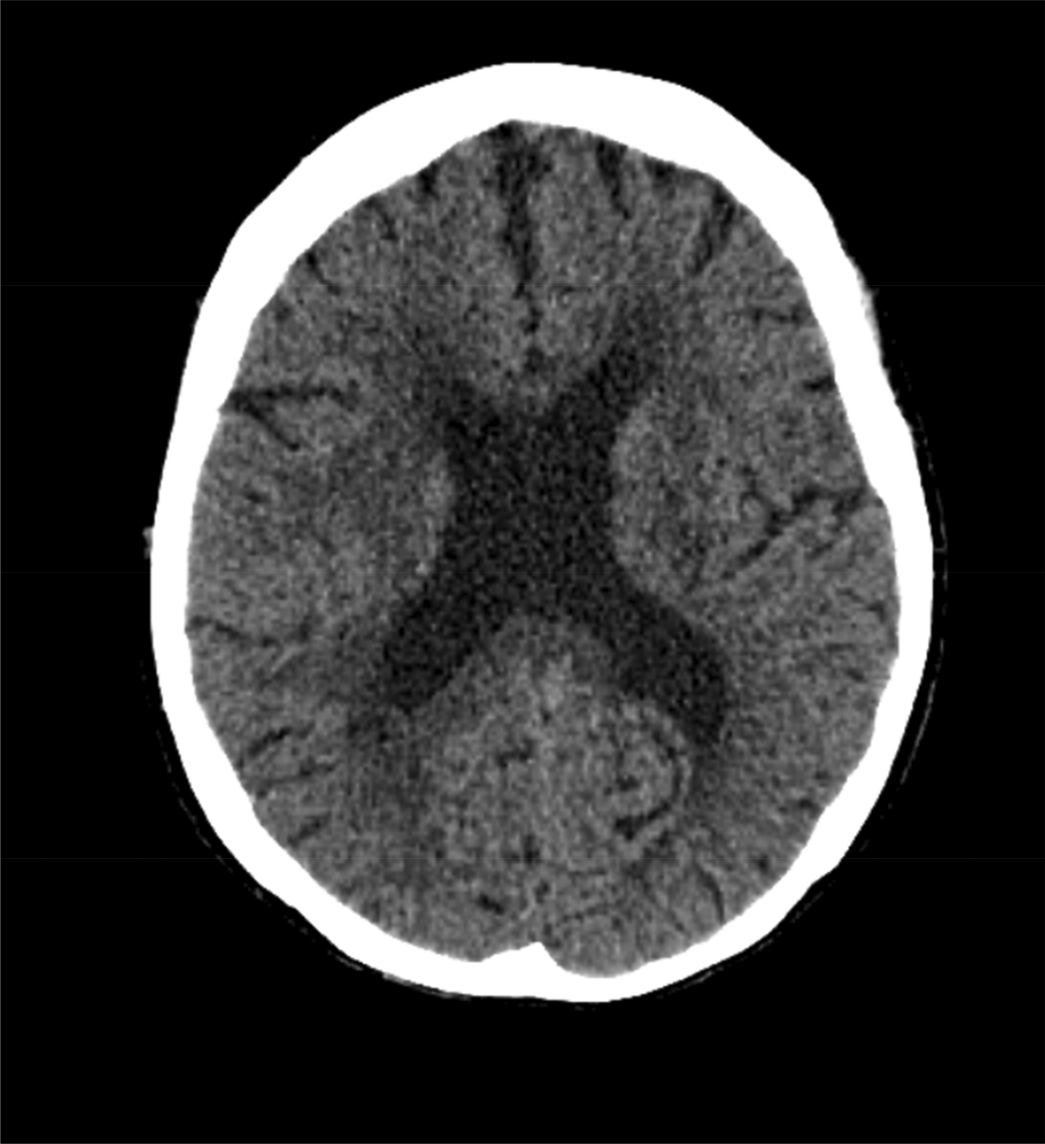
Initial computed tomography (CT) without contrast demonstrating no acute intracranial abnormality or hemorrhage or extra‐axial collections.
